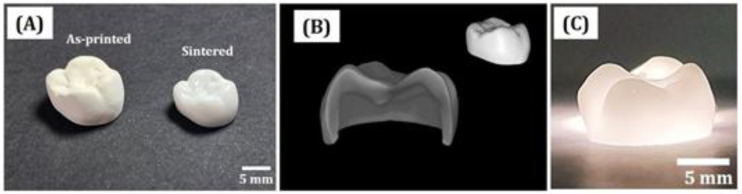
(A) Optical image showing 3D geometries of as-printed and sintered dental crowns, (B) representative μ-CT image of a sintered dental crown, and (C) optical image showing translucency of a sintered dental crown under light illumination.
